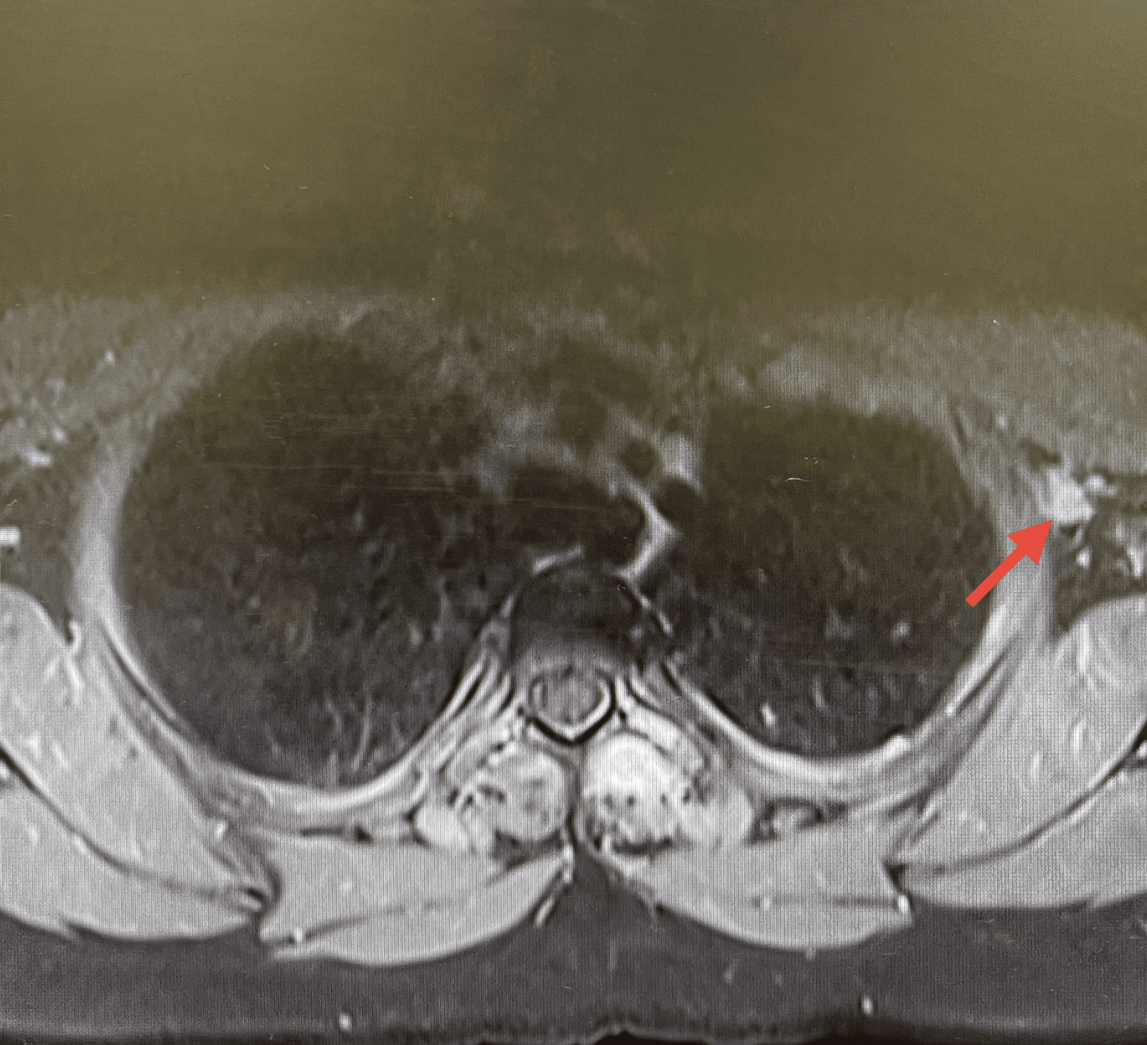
MRI showing enlarged left axillary lymph node (arrow), initially raising suspicion of metastatic disease.
